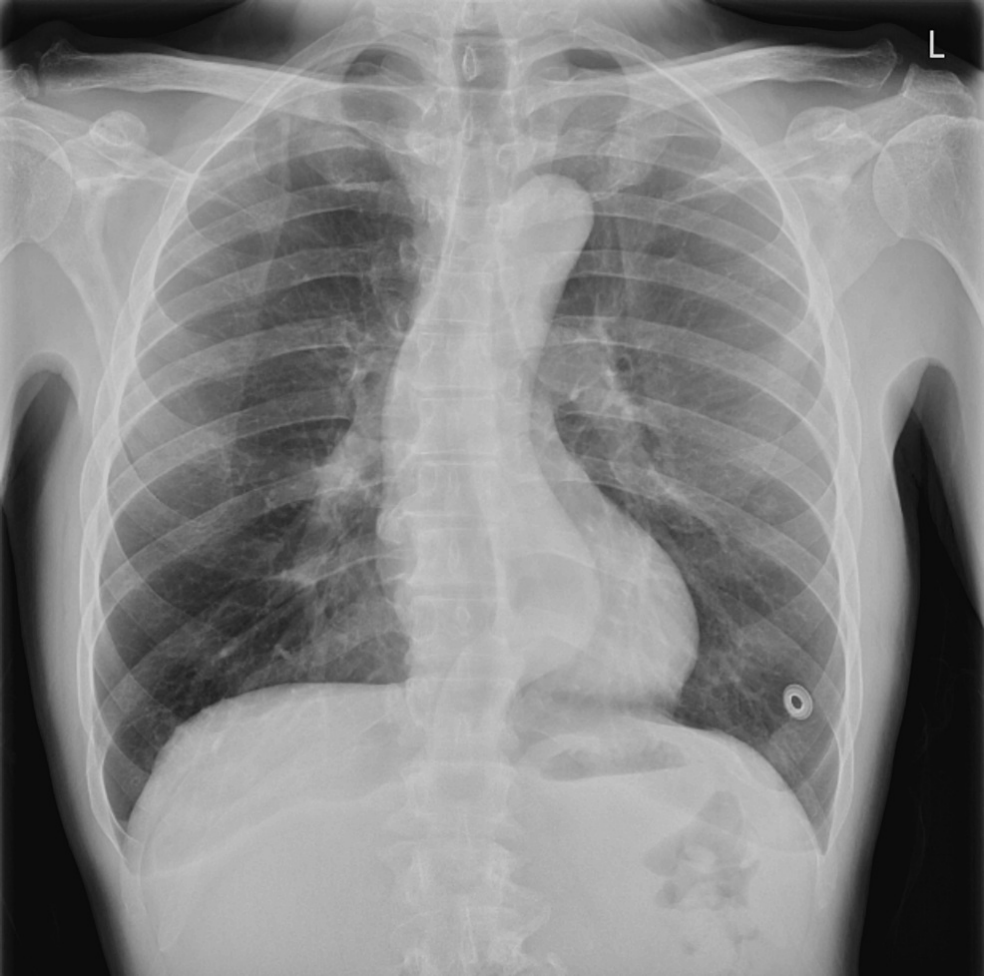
X-ray of chest at the time of admission showing both the lung fields equally inflated and clear. The hila, mediastinum, bony thorax, and pleura appear normal. The costo-phrenic and cardio-phrenic angles are clear.
